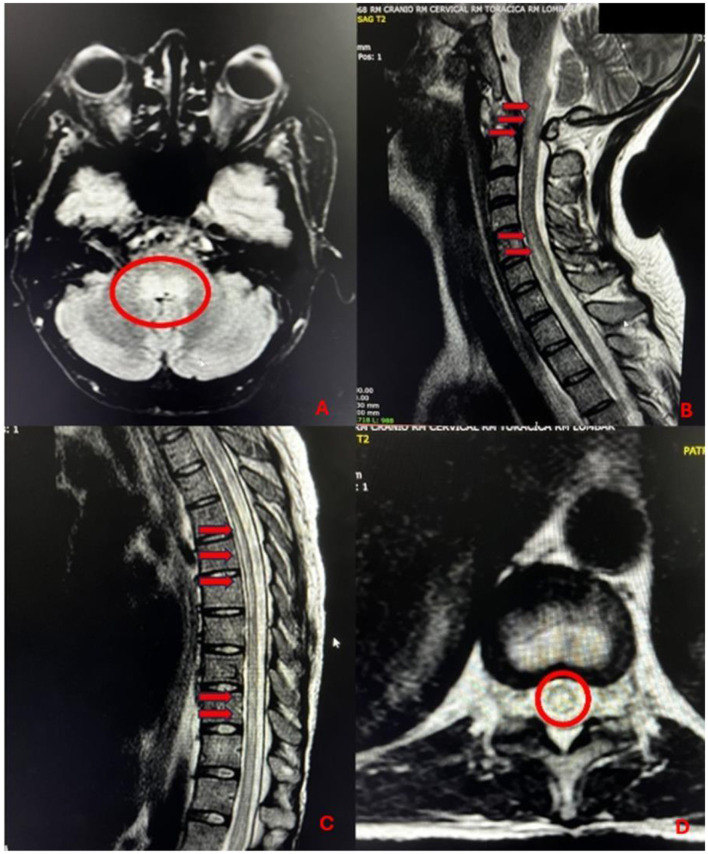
(A) T2 and FLAIR hyperintensity in the dorsal region of the pons, medulla oblongata and periependymal region of the fourth ventricle (red circles). (B) Isolated hyperintensive amorphous foci on FLAIR in the right semi-oval center, discontinuous signal changes in the central spinal cord, characterized by T2 hyperintensity from the pons to C2, and from C4 to C7, with slightly increased cord caliber in the latter. (C, D) Lower thoracic cord and conus medullaris displaying areas of signal change, characterized by T2 hyperintensity (red arrows).
